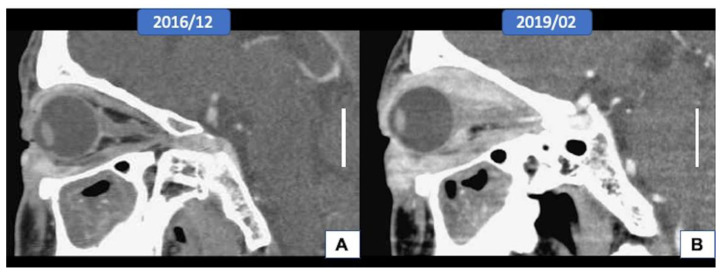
Radiological features of the tumor (scale bar, 2 cm). (A,B) The orbital computed tomographic scan showed the extension of the tumor from the eyelid to the whole orbital socket after three years.
